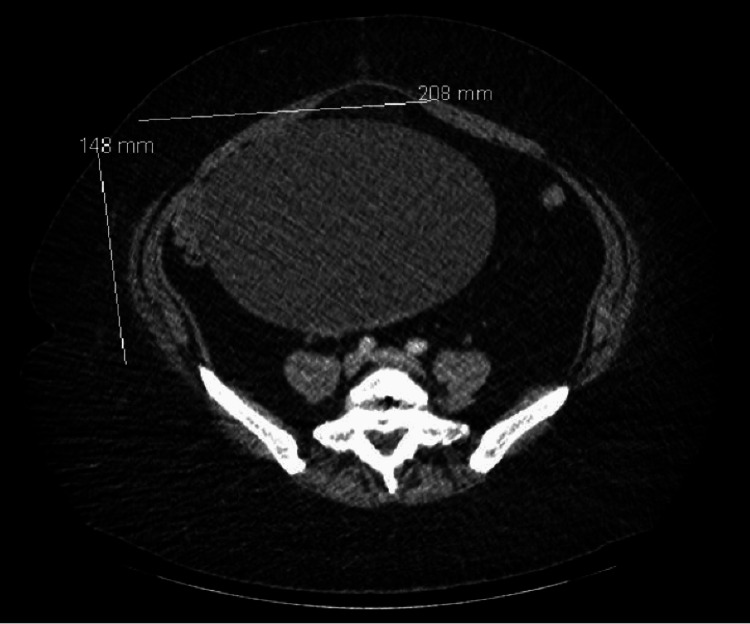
Abdominal CT scan showing a large cystic mass measuring 20.8 x 14.8 cm located in the right adnexal region
